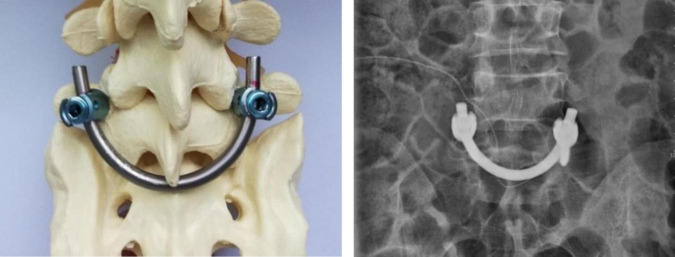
SFR shown on the model and X-ray. Two universal screws were inserted. A rod with a diameter of 5.5 mm was bent into a U-shaped rod and placed below the root of the spinous process of the L5. The rod end was connected and fixed with an ipsilateral pedicle screw. The screw holder was used to pressurize the broken end of the isthmus and then tighten the screw cap.
